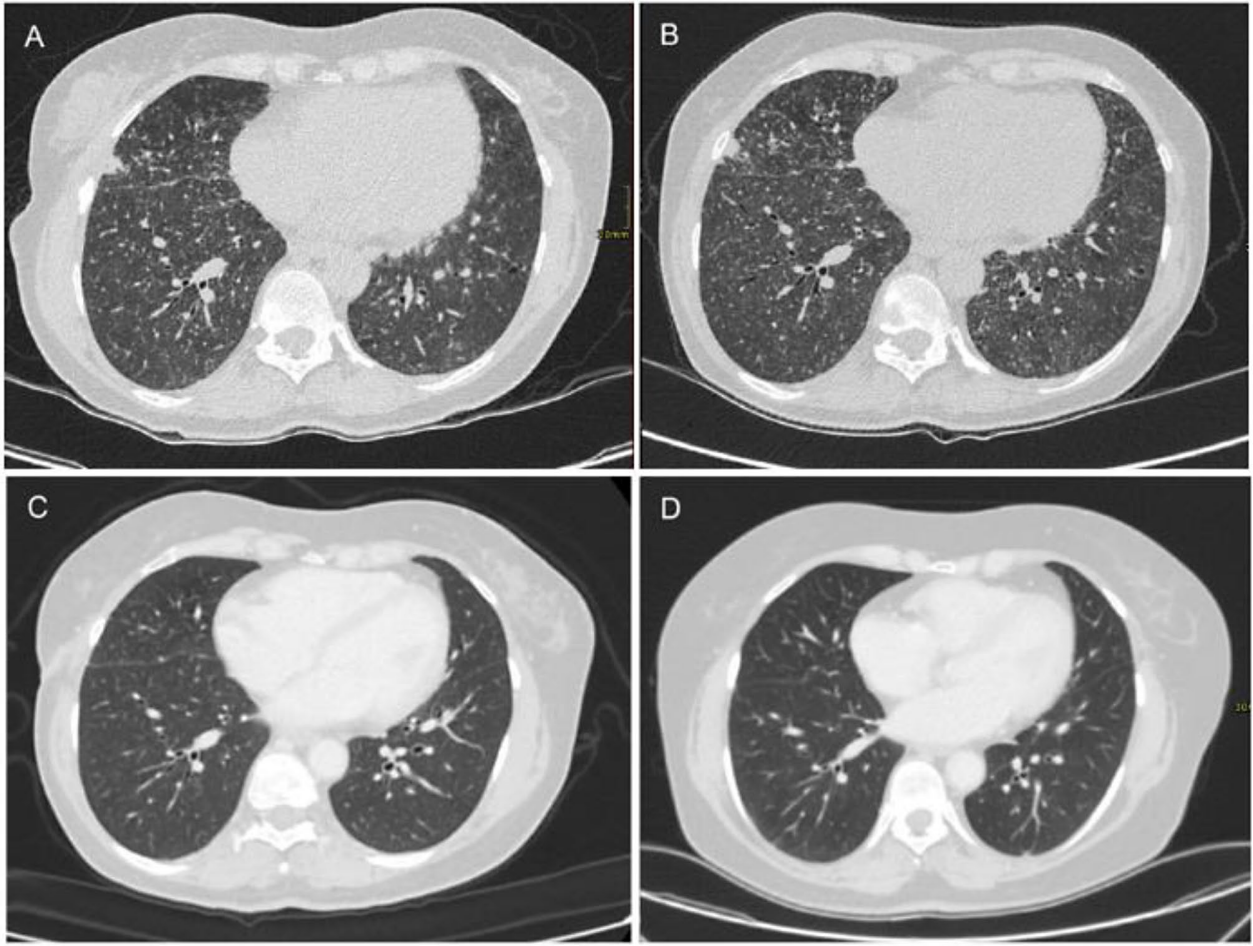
Serial computed tomography scans showing progressive resolution of the diffuse micronodular interstitial pattern of the lungs following initiation of antiretroviral therapy on 16 November 2022 (A: 26 November 2021; B: 22 September 2022; C: 30 January 2023; D: 30 May 2024)
